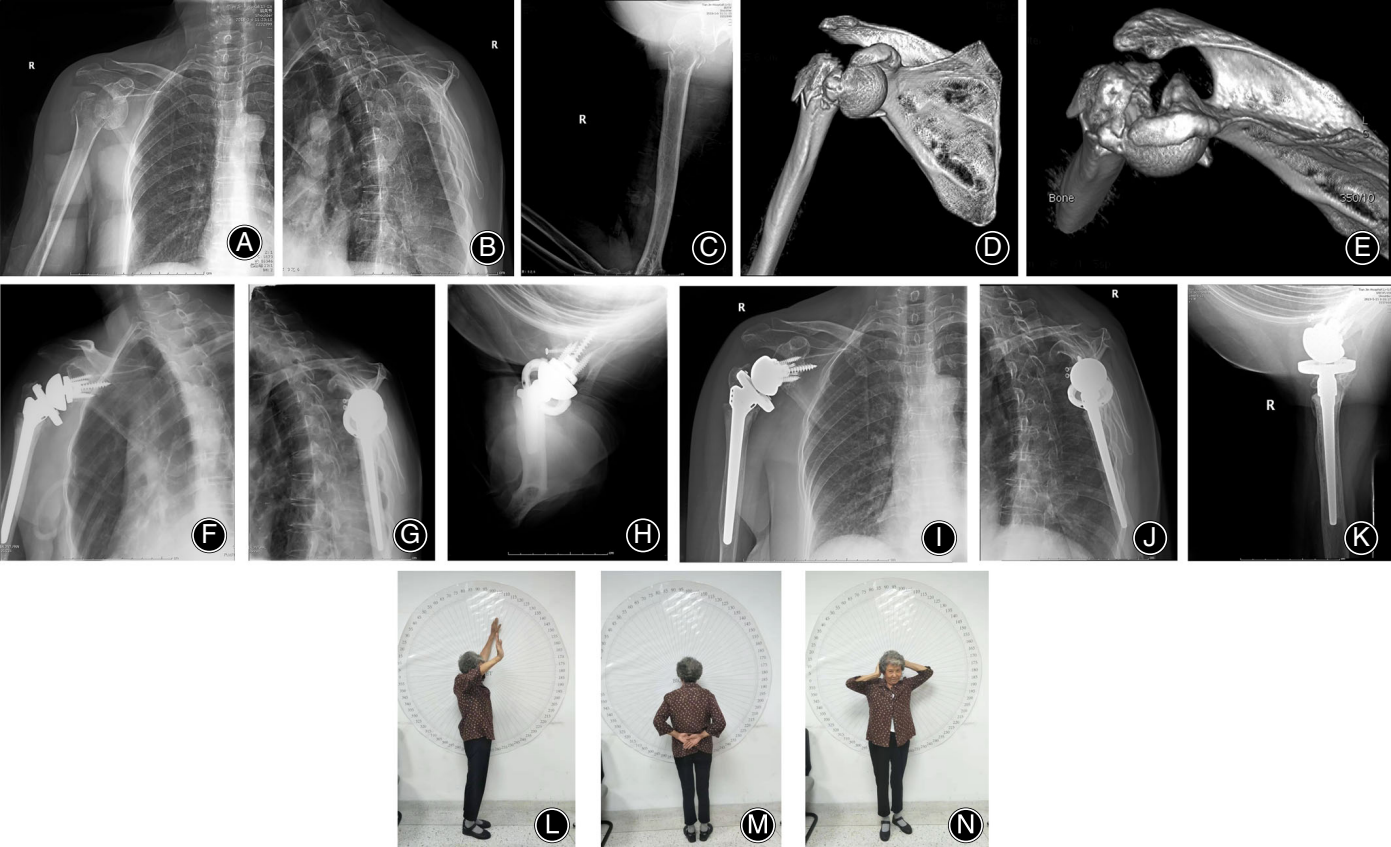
A 71 year‐old female patient with right proximal humeral comminuted fracture dislocation (Neer four part fracture dislocation) caused by a fall. (A–E). X‐rays and 3D‐CT showed comminuted fracture of proximal humerus with dislocation of glenohumeral joint. (F–H). The X‐rays in the AP view, Y view and axillary view indicated that the prosthesis position was satisfactory after the reverse shoulder arthroplasty. (I–K). At the end of 14 months follow‐up after operation, the X‐ray films of the AP view, Y view and axillary view showed satisfactory healing of the greater and lesser tuberosities. (L–N). At the end of 14 months follow‐up after operation, the forward elevation was 125°; external rotation was 35°; internal rotation was 50°. VAS score was 0°; The Neer score was 93; Constant‐Murley score was 83.
